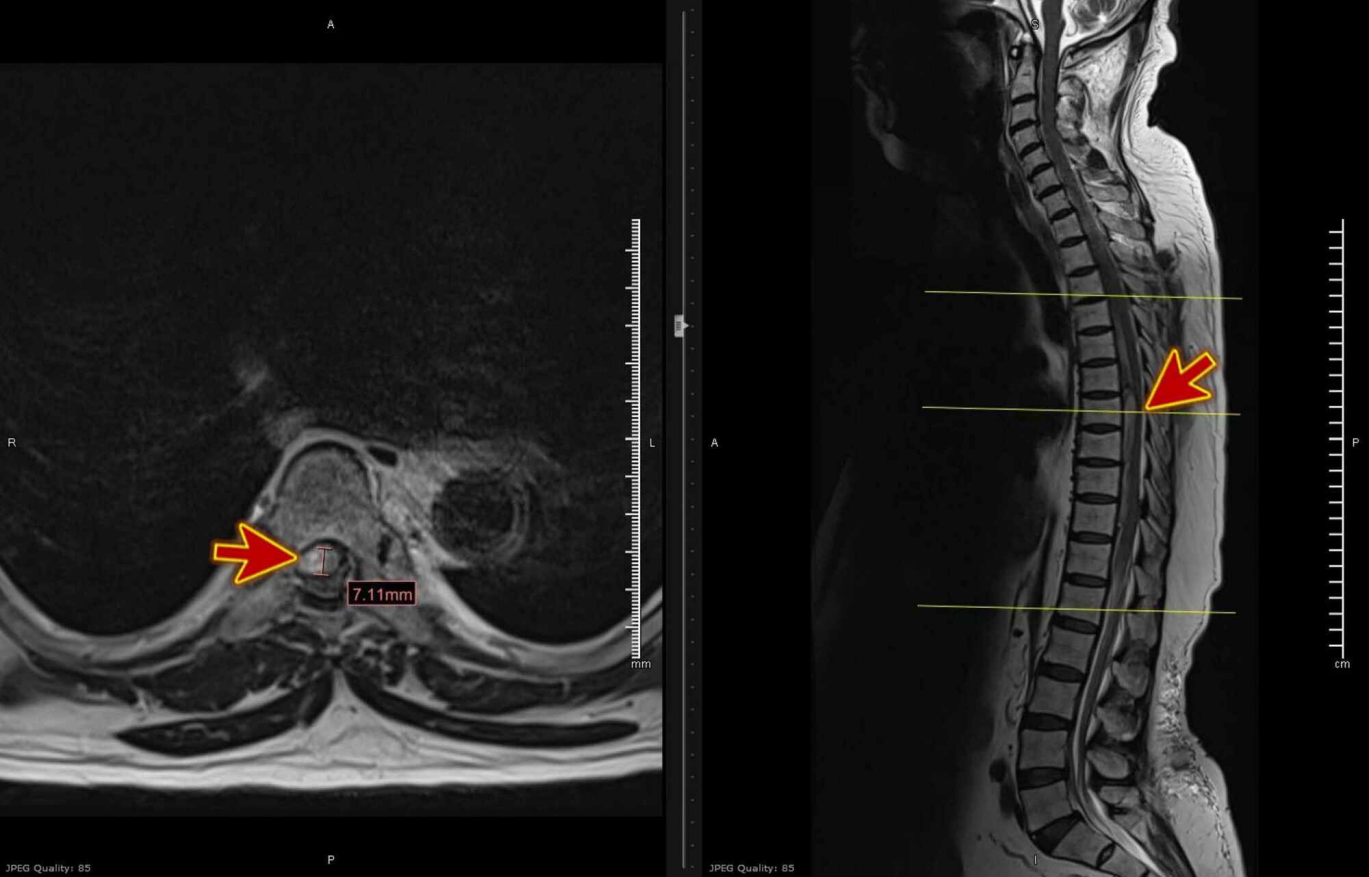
MRI T2WI axial view shows extensive extradural hematoma severe mass effect on the cord (red arrows), most severe at the level from the seventh to the tenth dorsal vertebra (D7-D10) MRI: magnetic resonance imaging, T2WI: T2-weighted image.
